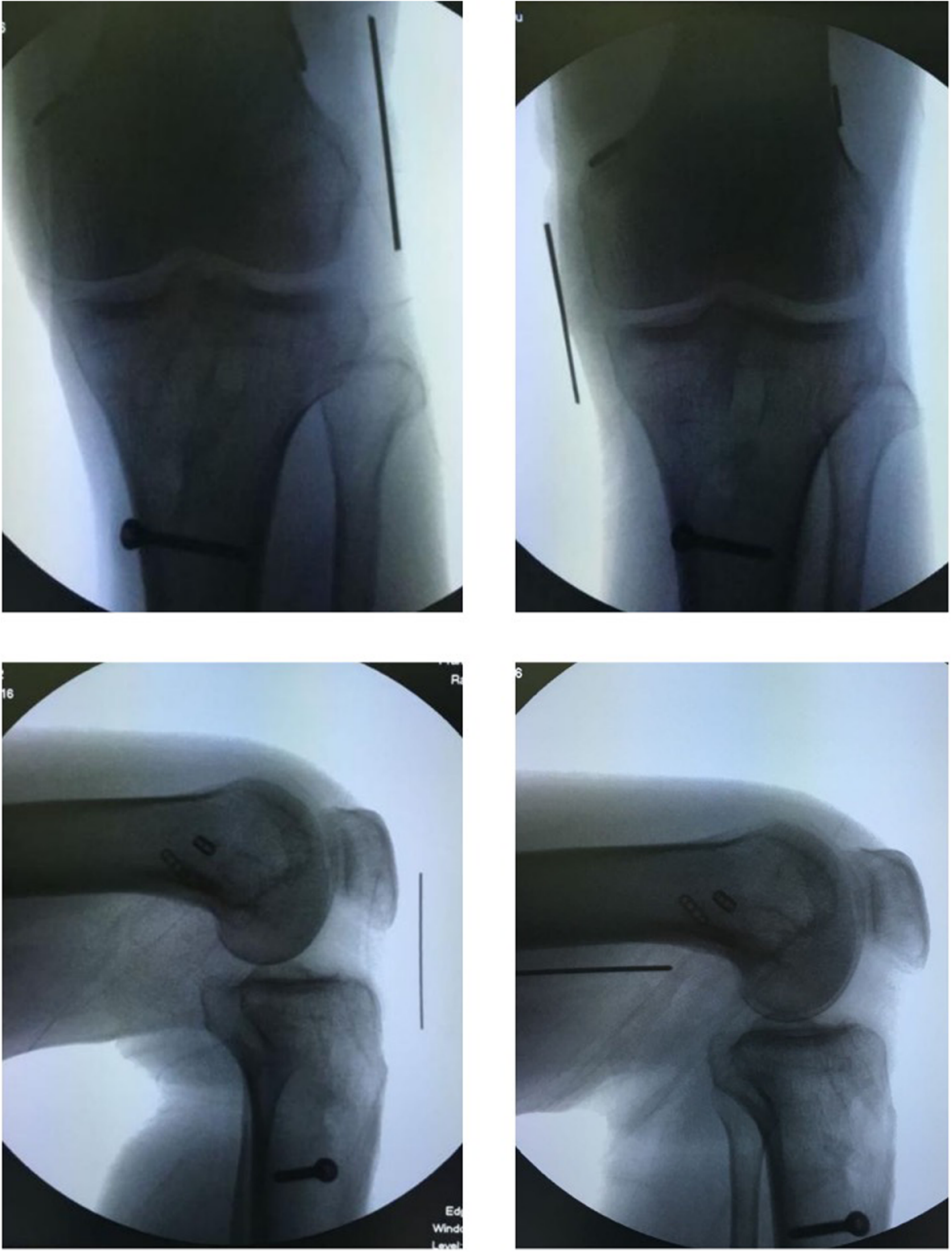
The postoperative X-rays of a 45-year-old man with PMC injury
